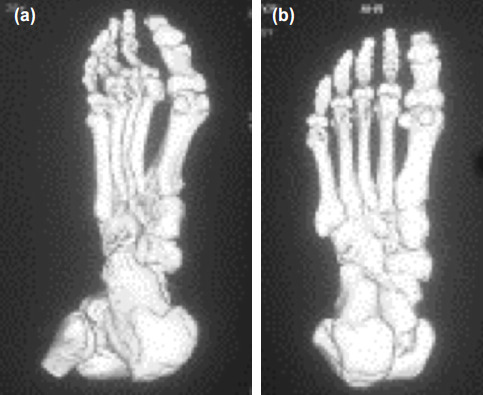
The shaded surface display on CT scan showing a good plantar contour of the second metatarsal; (a) oblique view and (b) inferior view.
